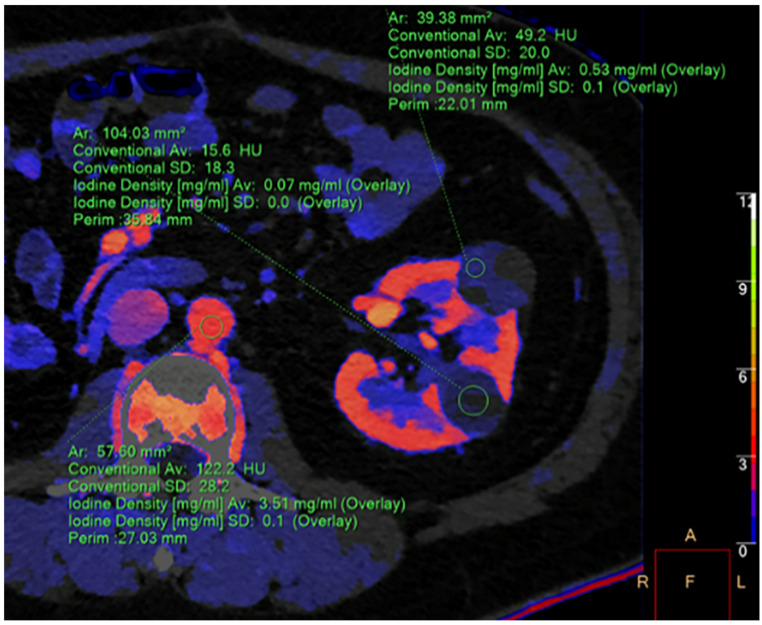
In the contrast-enhanced iodine map CT, iodine-containing pixels are assigned values equal to the concentration of iodine, expressed in mg/mL [2]. The image shows a 2.5 cm inhomogeneous hypovascular anterior renal mass (iodine concentration 0.57 mg/mL) consistent with papillary renal cell carcinoma and a 2.2 cm avascular posterior renal mass (iodine concentration 0.07 mg/mL) representing a simple cyst. A third region of interest in the abdominal aorta shows an iodine concentration of 3.5 mg/mL.
